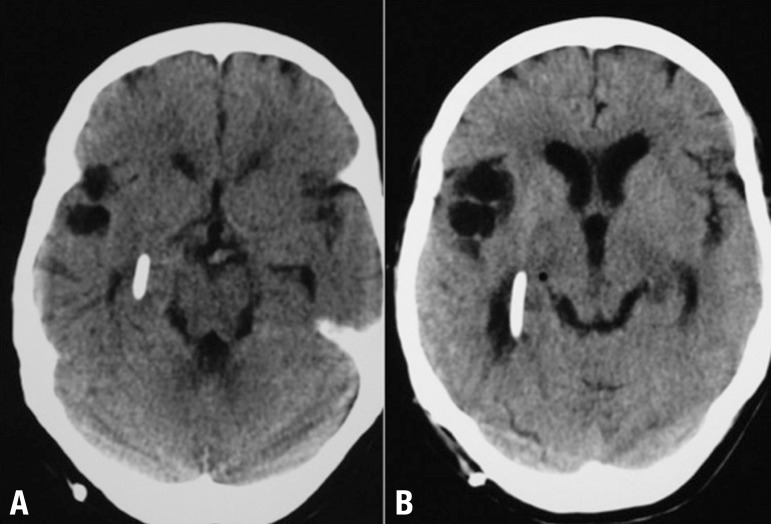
[A] Noncontrast brain CT-scan after first shunt derivation shows catheter
